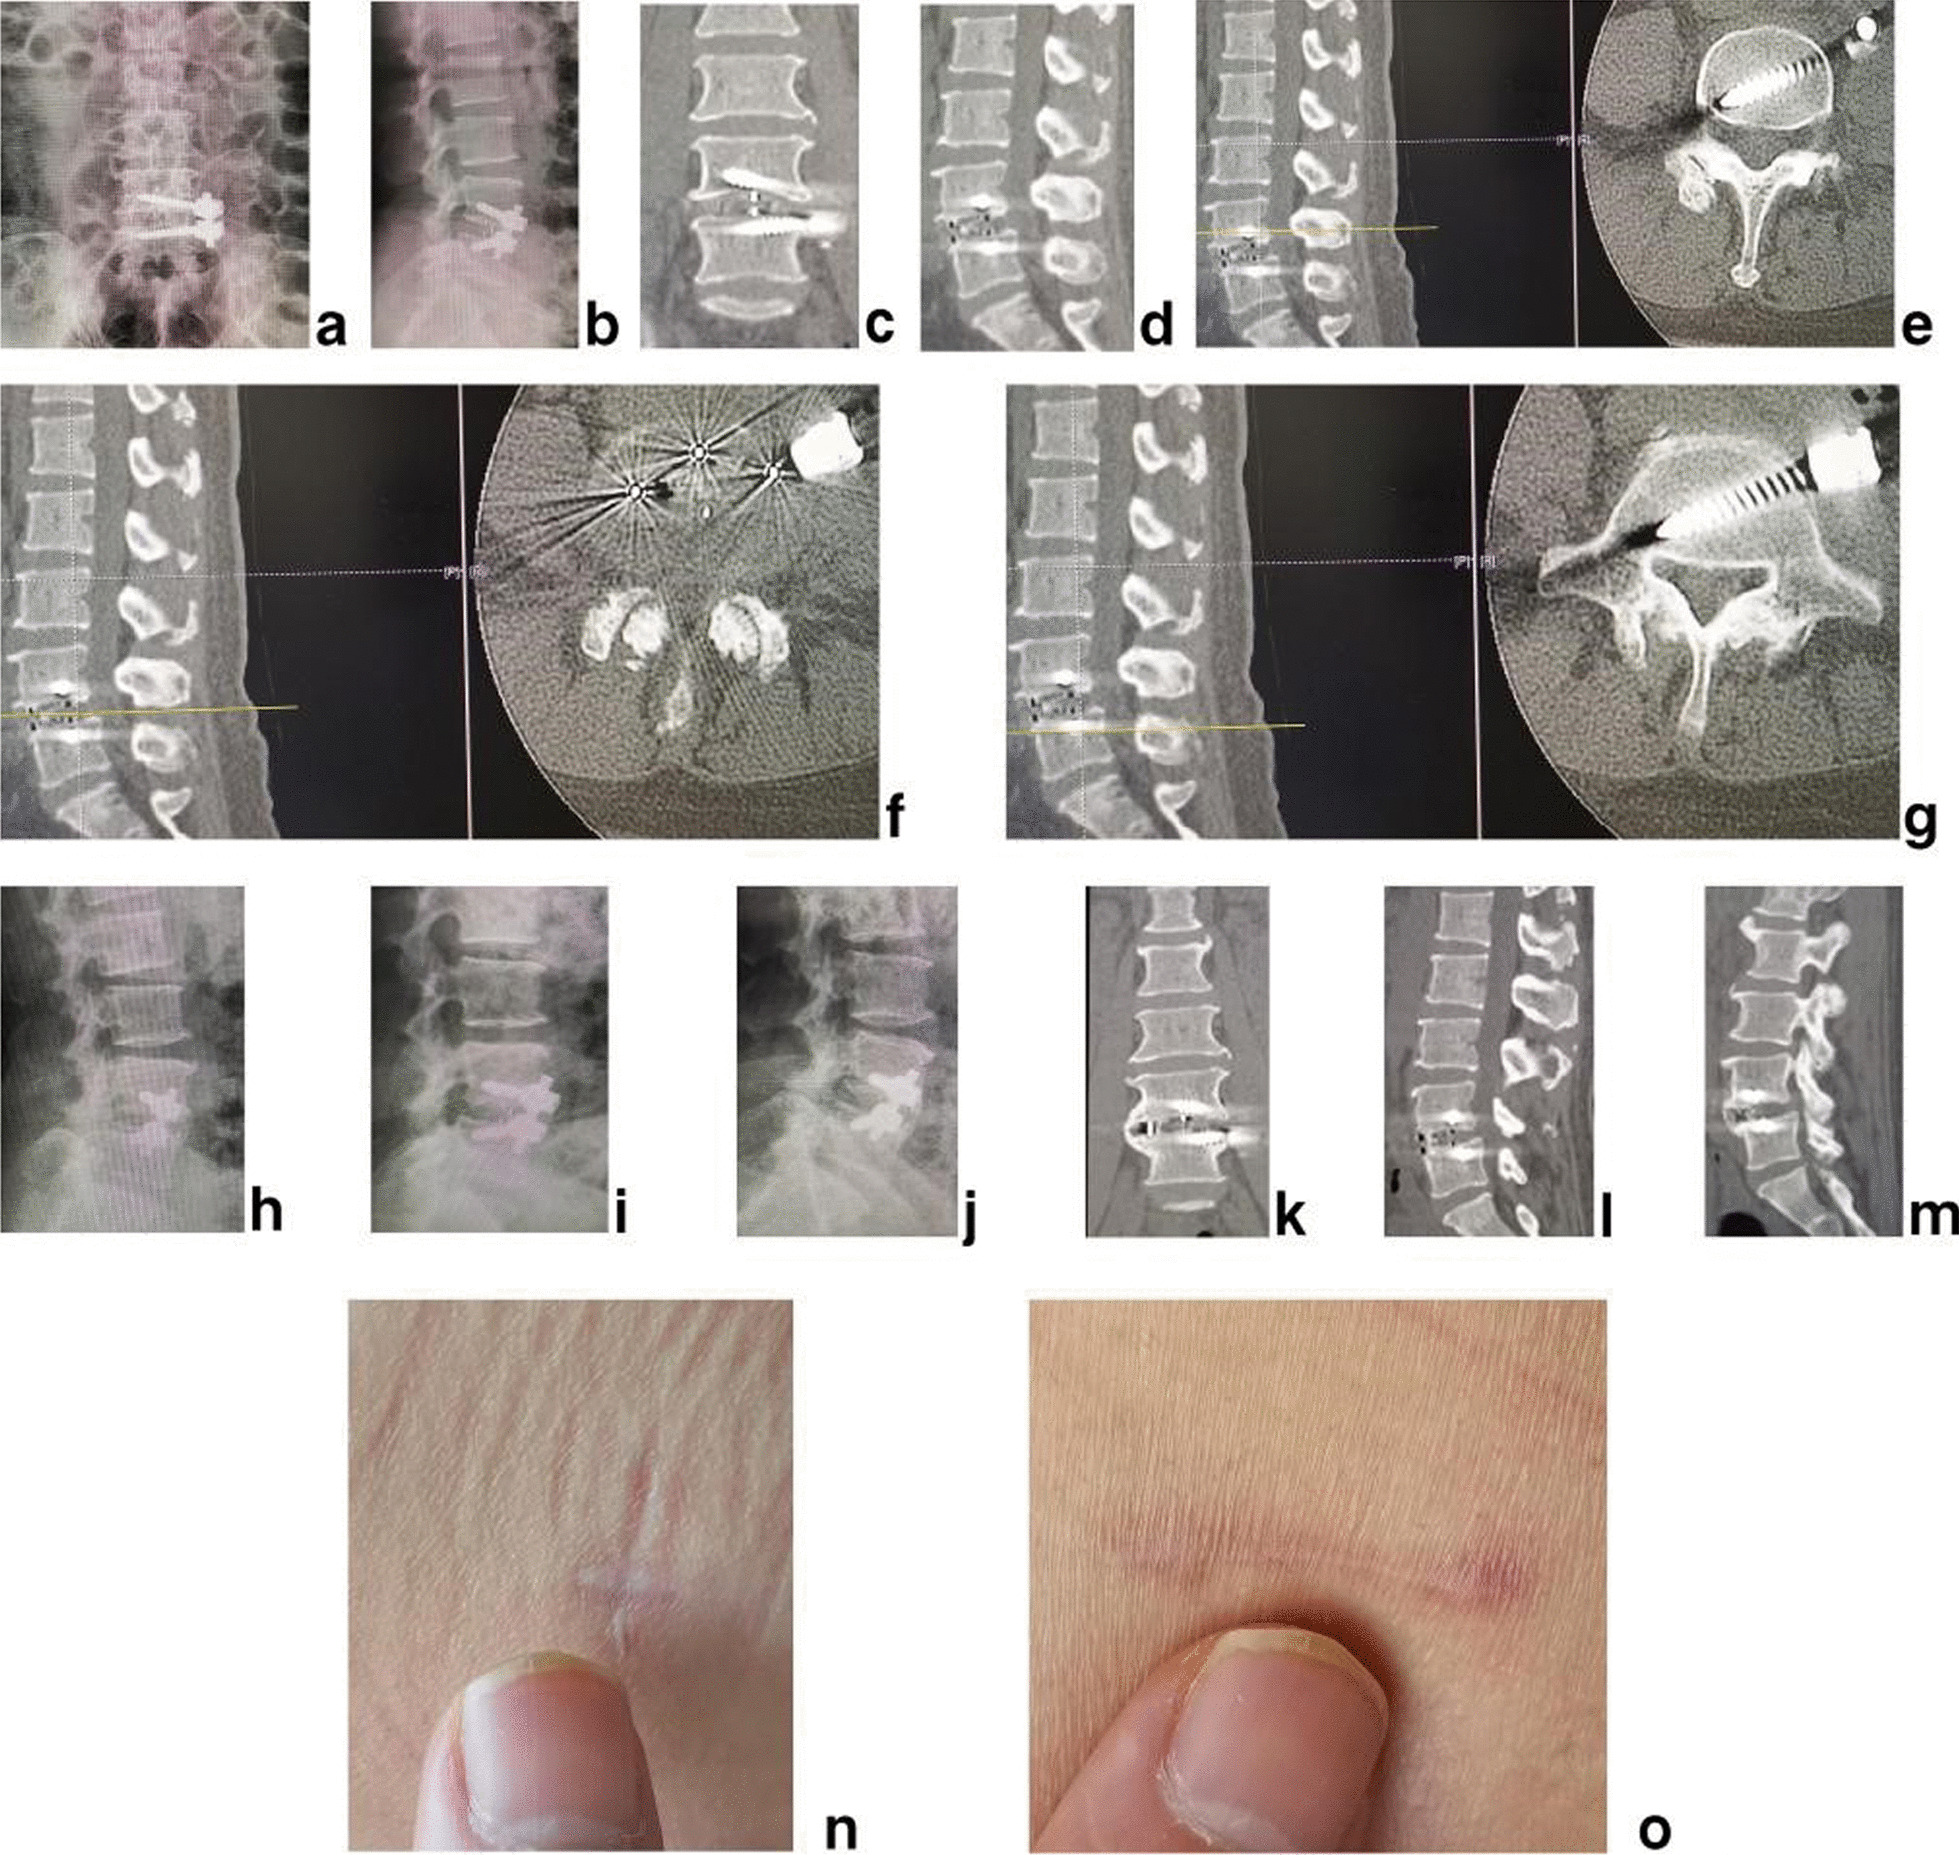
a Posteroanterior and b lateral X-ray image, c coronal, d sagittal and e–g axial CT image showed good postoperative position of cage and screws immediately after operation. On h lateral X-ray image there were no significant changes in intervertebral space height, lumbar lordotic angle and operative segmental lordotic angle 2 years after operation. No instability at fusion level was found on i hyperflexion and j hyperextension lateral X-ray image. Fusion grade at 2-year follow-up was grade I on k coronal and l, m sagittal CT images. The picture shows the cosmetic incisions for n PTES and o OLIF 2 years after operation
